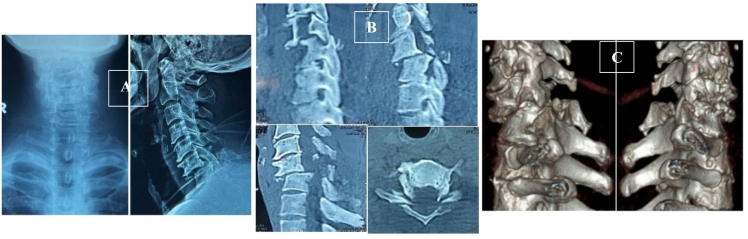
Pre-operative Cervical (A) plain x-ray and CT-Scan of (B) sagittal view, axial view, and (C) 3D view showed compression fracture on C7 and bilateral facet dislocation on vertebrae C6-C7 associated with bi-laminar fracture of C6.
